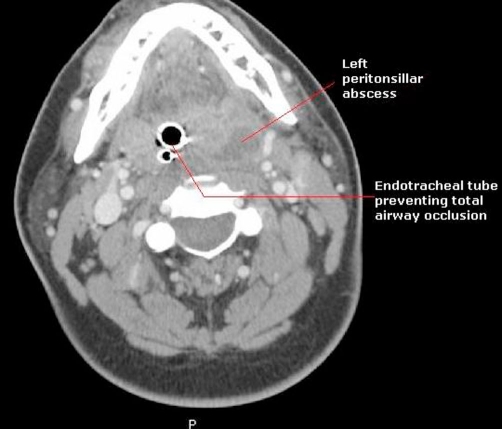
Computer tomography (CT) scan of neck. Left peritonsillar abscess causing airway occlusion. Total airway occlusion is prevented by an endotracheal tube.
